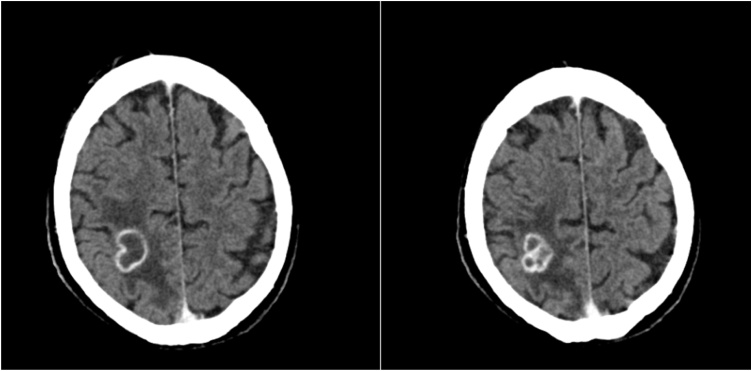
CT Scan with contrast with a 2.5 cm lesion at the right upper parietooccipital region.
